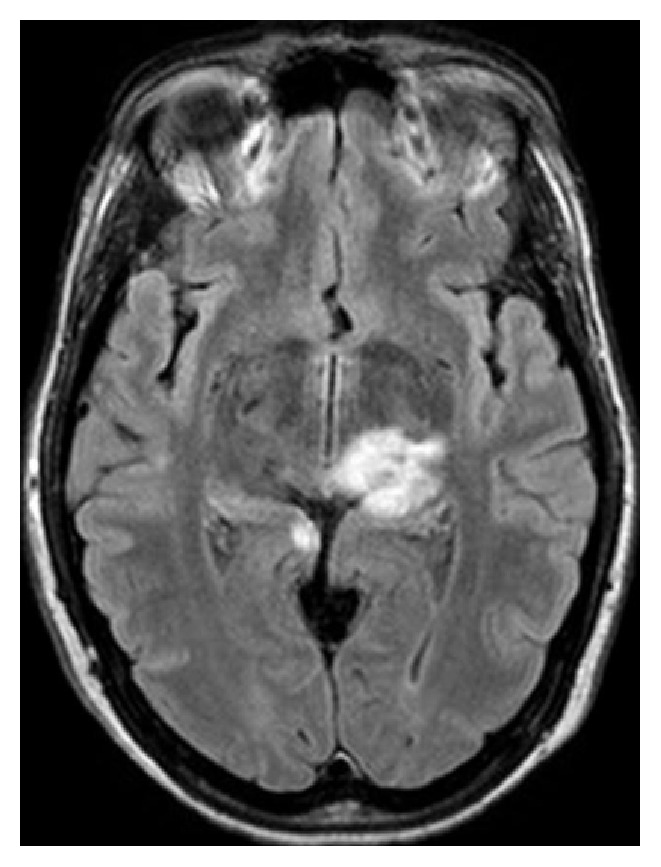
MRI brain T2 axial FLAIR sequence showed hyperintensities in the left thalamus and right mesial occipital lobe.
